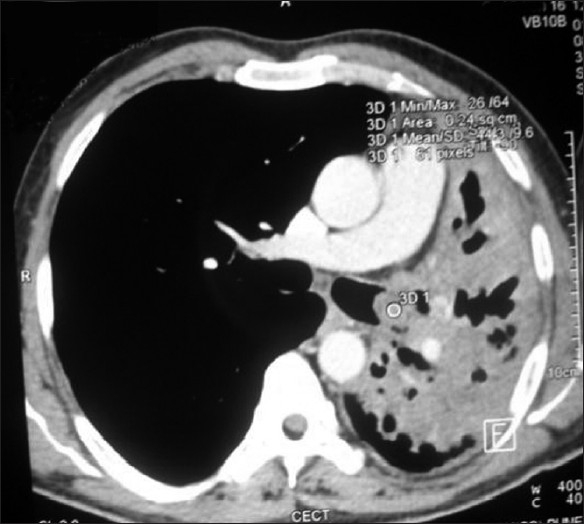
CT scan of chest showing endobronchial mass obstructing left main bronchus and collapse consolidation of left lung
